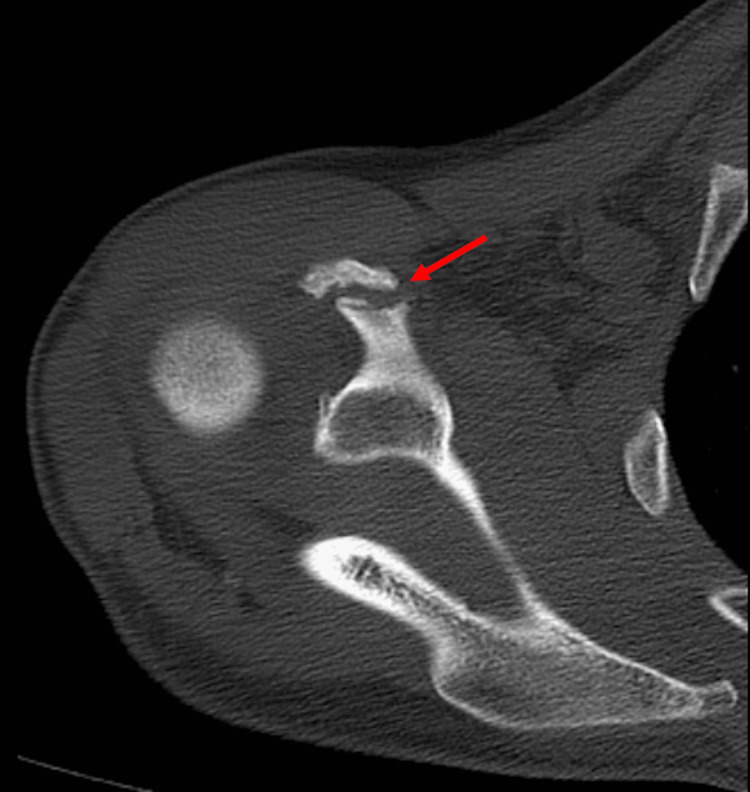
Axial CT scan of the right shoulder (Case 1) The red arrow shows coracoid non-union. CT: computed tomography
